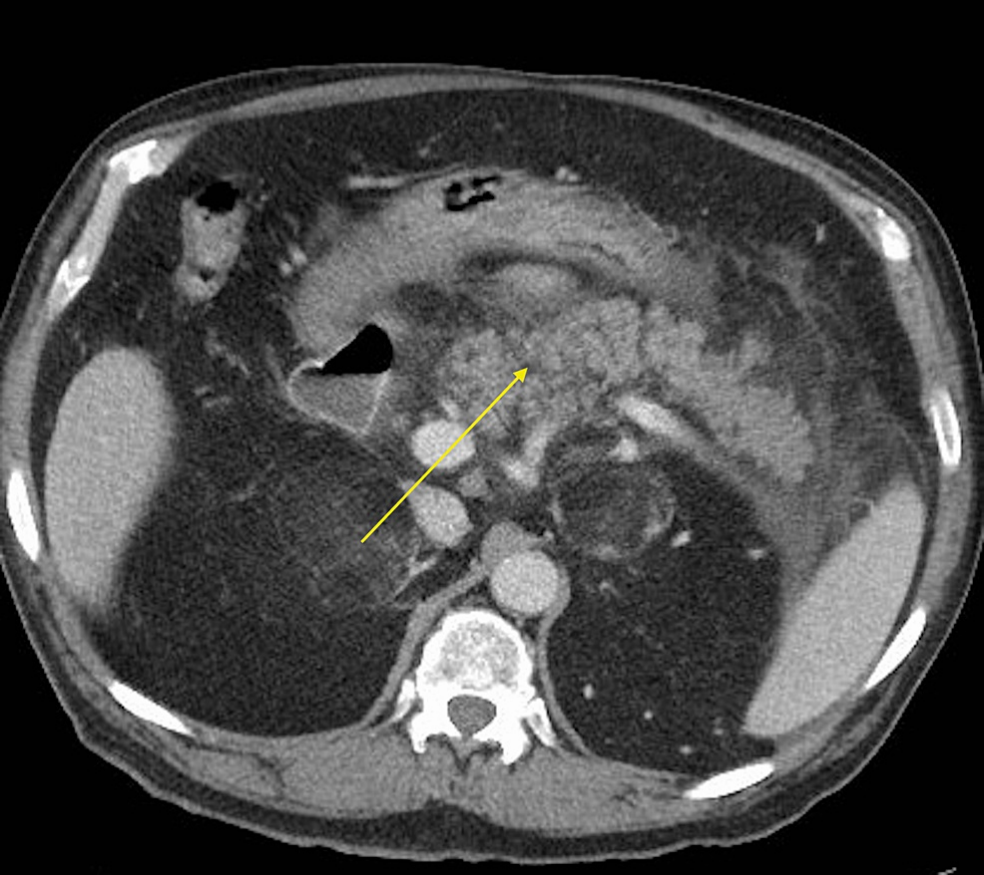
CT scan of abdomen and pelvis with contrast. Arrow shows diffuse edematous inflammation of pancreatic head, body, and tail
